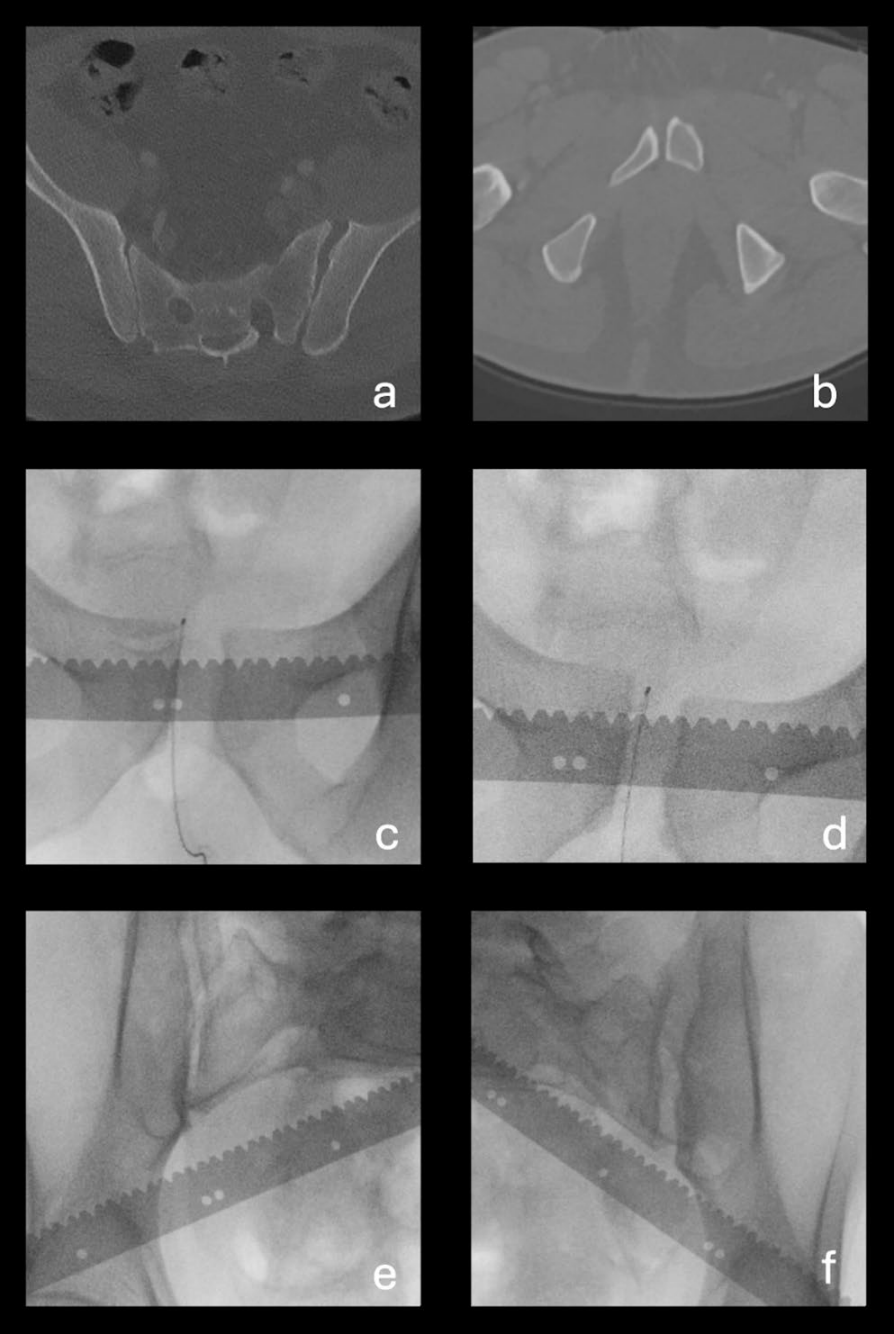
Case 29 is an example of a case when there is substantial sacroiliac (SI) joint diastasis, which is not reflected by the measurements of displacement in the anterior pelvis. (a) axial CT scan of the sacrum with pelvic binder on, showing diastasis in the left SI joint (b) axial CT image of pubic symphysis showing minimal symphyseal diastasis (c) anterior-posterior (AP) examination under anaesthesia (EUA) image of the pubic symphysis in the external rotation stress maneuver, showing 1.6 cm of symphyseal diastasis (d) AP EUA image of the pubic symphysis in the push/pull stress maneuver, showing 0.7 cm of rotational instability (e) Obturator inlet EUA image of right SI joint in the external rotation stress maneuver, demonstrating no SI diastasis (f) Obturator inlet EUA image of left SI joint in the external rotation stress maneuver, showing complete SI diastasis
